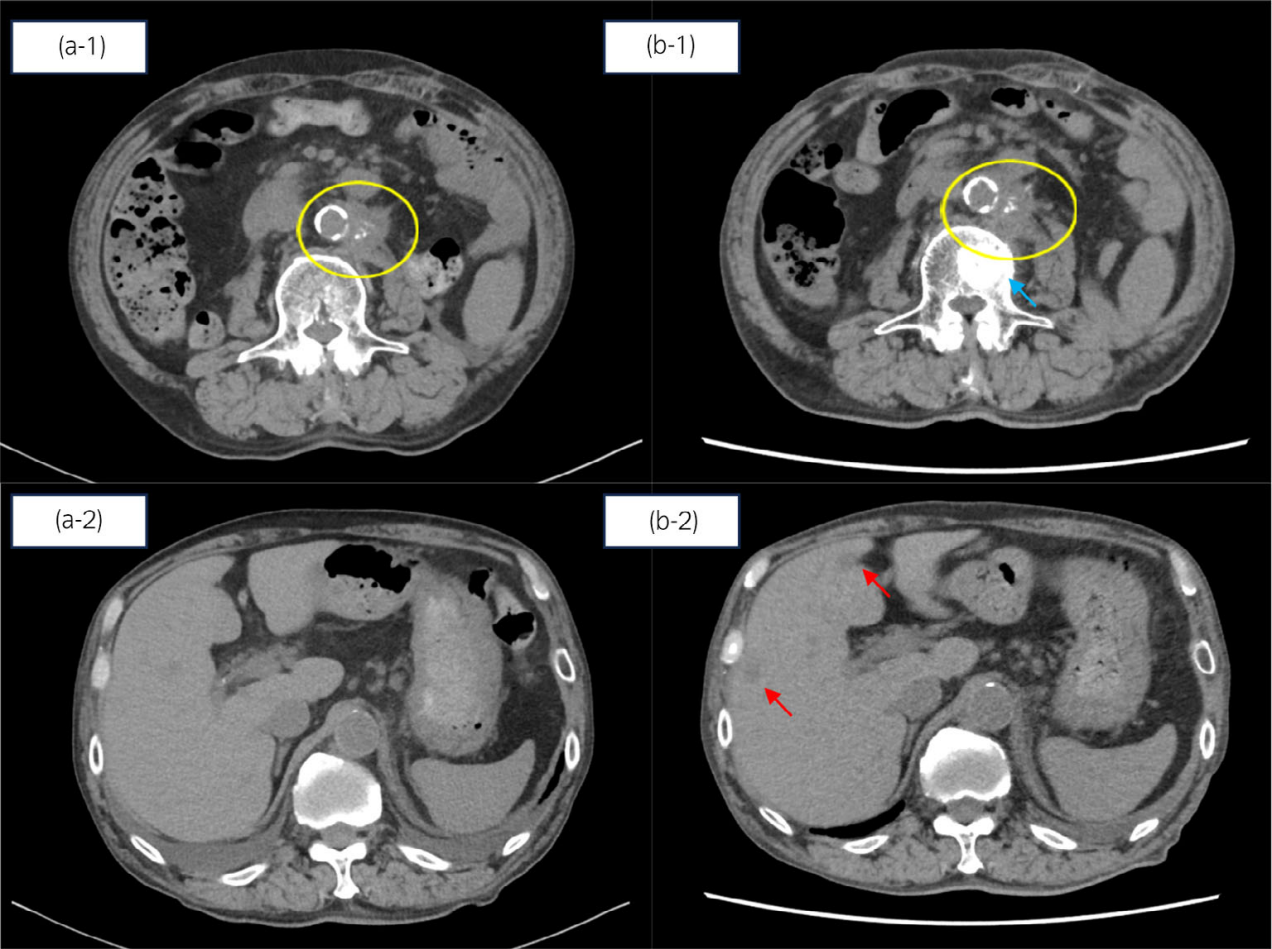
CT findings of Patient 2. CT from December Y‐1 before EV treatment demonstrating increase in size of para‐aortic lymph node (yellow circle, a‐1), normal lumbar vertebrae (a‐1), and normal liver (a‐2), and from March Y after two cycles of EV demonstrating progressive disease (PD), which is an increase in the size of para‐aortic lymph nodes (yellow circle, b‐1) and metastasis that appeared in the lumbar vertebrae (blue arrow, b‐1) and liver (red arrow, b‐2).
